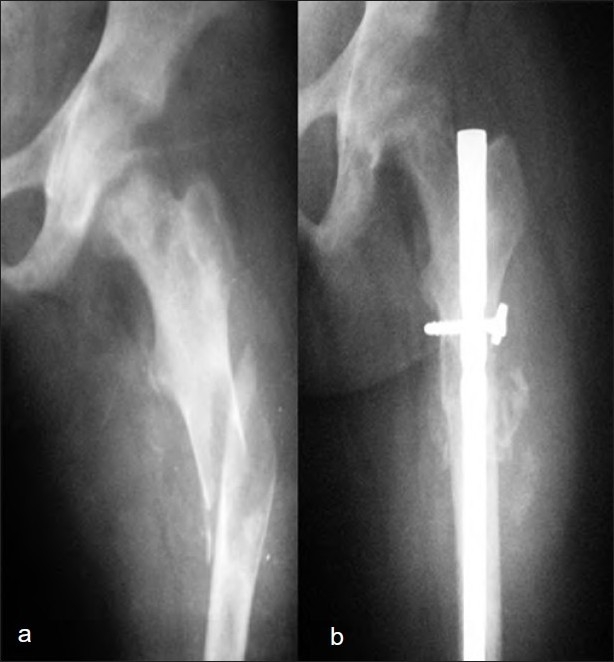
(a) Anteroposterior radiograph of femur showing lytic area with pathological fracture shaft femur. (b) At 1 year follow up radiograph showing union and nail *in situ*
